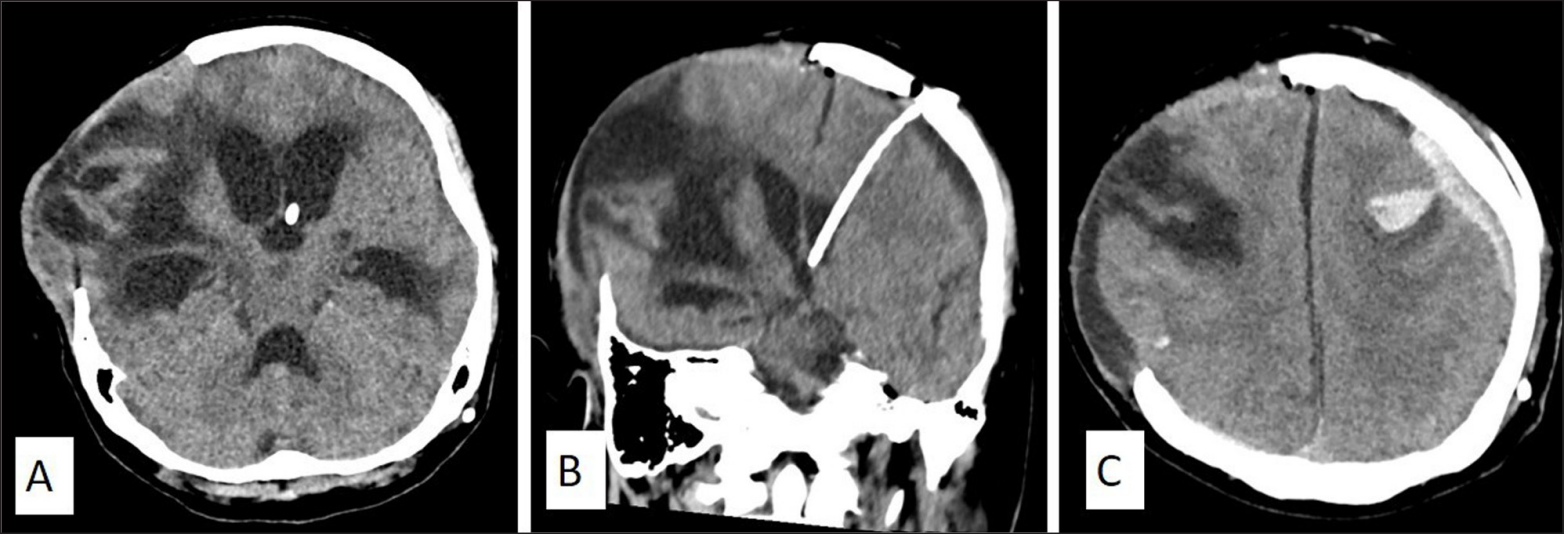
Axial brain CT of a 45-year-old woman who had right decompressive hemicraniectomy for evacuation of a right basal ganglia hemorrhage. She developed hydrocephalus for which a left frontal external ventricular catheter was inserted (A). Coronal CT (B) shows post catheterization improvement of the hydrocephalus (B). During removal of the ventricular catheter, the procedure was complicated by development of an acute left subdural and intraparenchymal hemorrhage along the catheter track seen on the axial CT (C).
